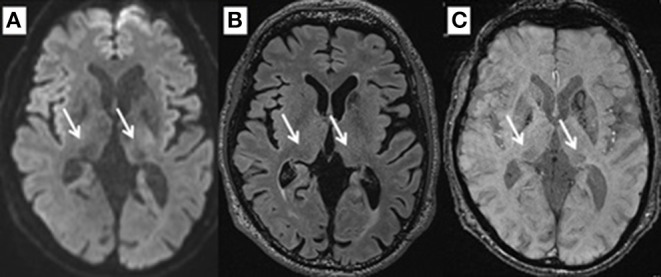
Pulvinar hypointensity MRI of a 61-year-old man in DWI (A), FLAIR (B), and SWI (C).
